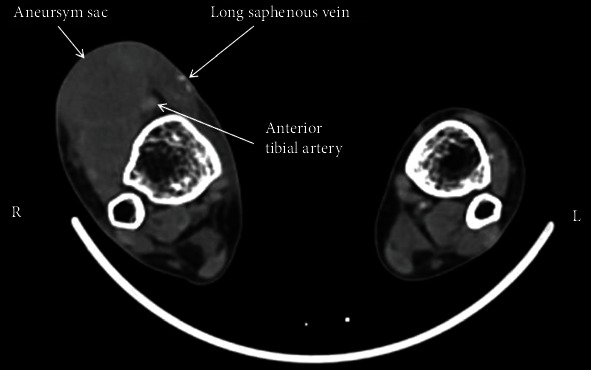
Computed tomography of the patient's right ankle aneurysm shows calcification in the saphenous vein and an intact aneurysm.
